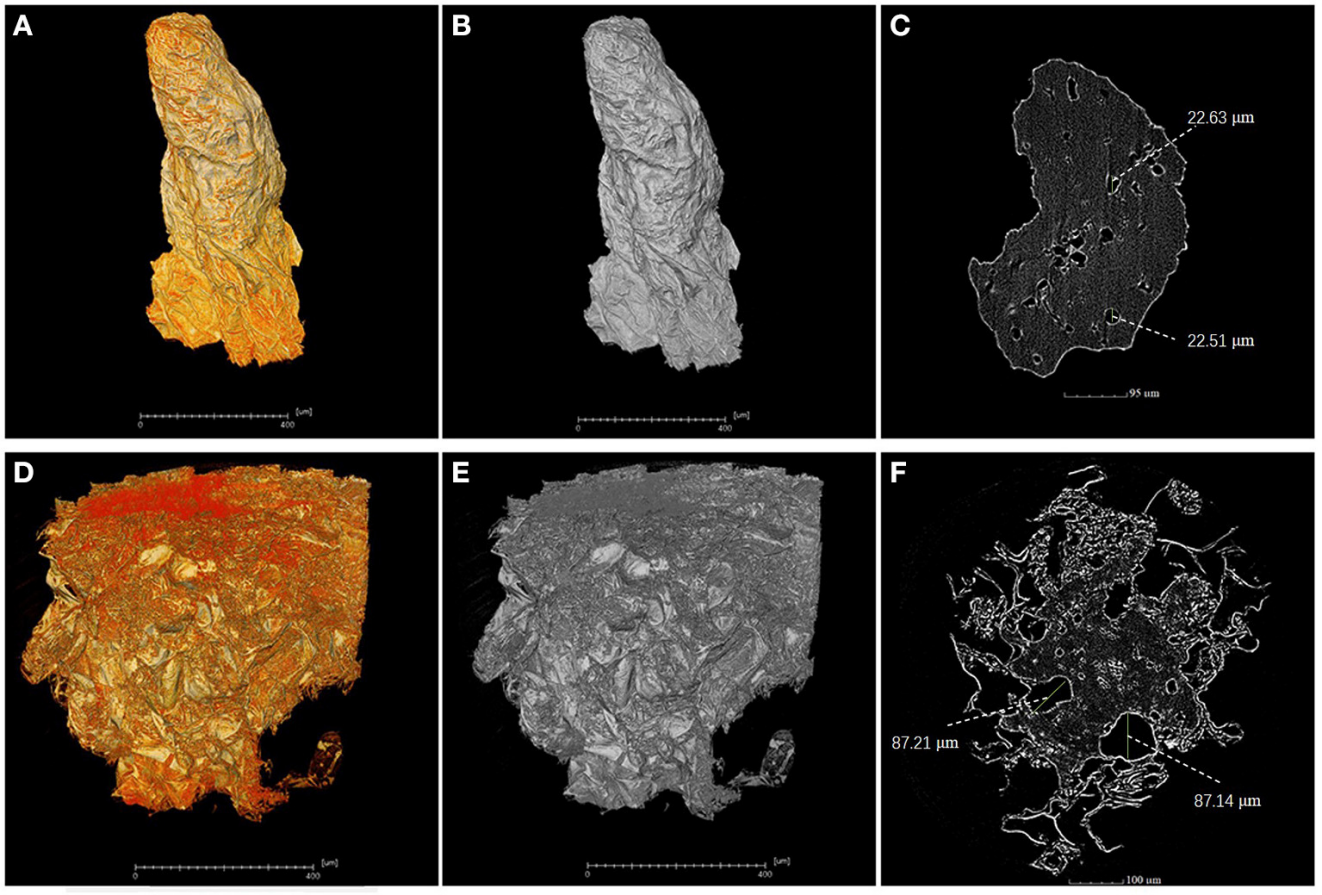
High-resolution analysis of flesh microstructure of “Akizuki” fruit. (A,B) Yellow and gray reconstructed 3D images of flesh from the MRA (middle-risk area with cork spot) of the healthy fruit, respectively. (C) Segment of the X-ray scanning of the healthy fruit flesh. (D,E) Yellow and gray reconstructed 3D images of flesh from the MRA of cork spotted fruit, respectively. (F) Segment of the X-ray scanning of the cork spotted fruit flesh.
